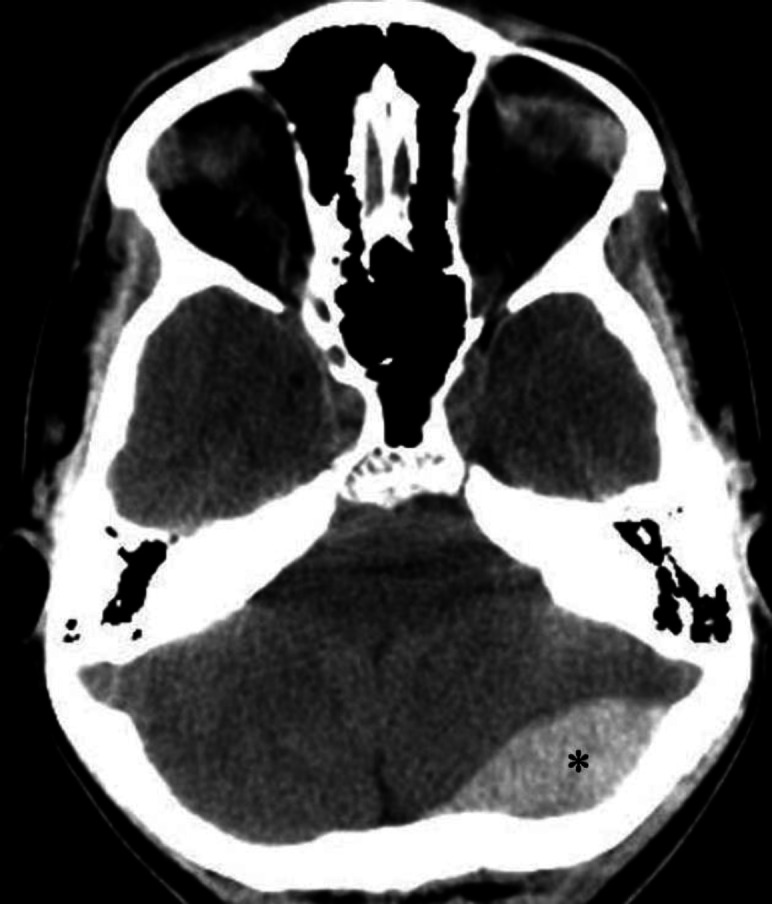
Non-contrast CT brain of an 18 year-old male with an epidural (extra-dural) hematoma. The hematoma (asterisk) appears as a sharply demarcated biconvex hyperdense area in the left occipital region. Note the mild left-to-right midline shift
